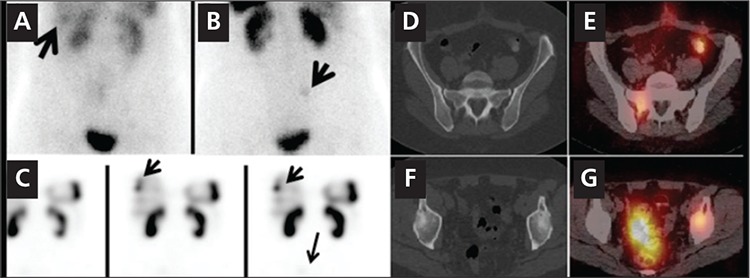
A 58 year-old woman with carcinoid tumor of the small bowel withknown liver metastases had an In-111 Pentetreotide scan. Anterior (A) andposterior (B) planar images of the abdominopelvic region show abnormal focaluptake is noted in the inferior right lobe of the liver (arrow, image A) and afaint focus of increased uptake is noted in the mid upper right hemi pelvis(arrow, image B). Selected coronal SPECT (C) images show foci of increaseduptake in the liver, corresponding to the known sites of liver metastases (smallarrows). A very faint focus of tracer uptake slightly above the bladder on theright is suspicious for a metastasis (arrow, C). However accurate interpretationof this finding is not possible with SPECT scan only. Axial SPECT/CT images atthe level of pelvis (D, E, F, G) show increased tracer uptake in the right sacrum(D,E), consistent with early bone metastasis, which correlated to the suspiciousfinding on planar posterior image (B). Another focus of abnormal increasedtracer uptake was noted in left acetabulum with predominantly scleroticchanges in the acetabulum (F, G). This lesion was not visible on planar imagesand was not localizable on SPECT alone. SPECT /CT however detected andlocalized this metastatic bone lesion
